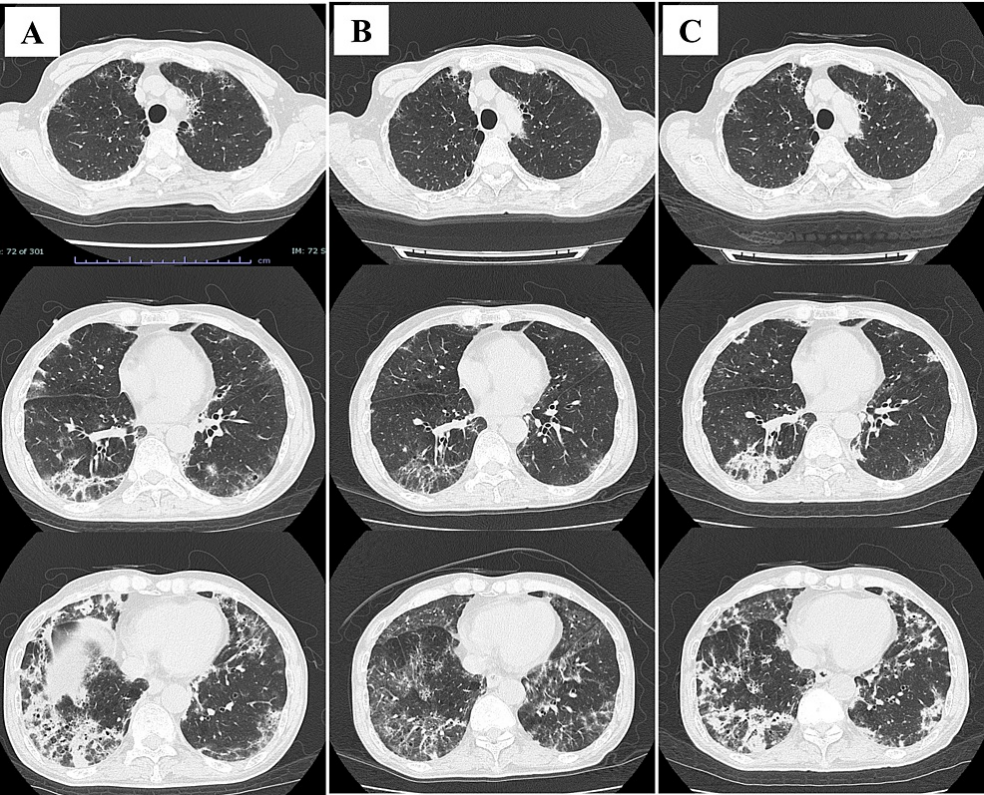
High-resolution computed tomography (HRCT), performed two days after hospitalization, revealed peripheral predominant consolidation with traction bronchiectasis and peribronchovascular thickening in the lower lobes (A). An HRCT performed 22 days after hospitalization indicated improvement in consolidation (B). An HRCT performed 35 days after hospitalization showed re-enhancement of the consolidation (C).
